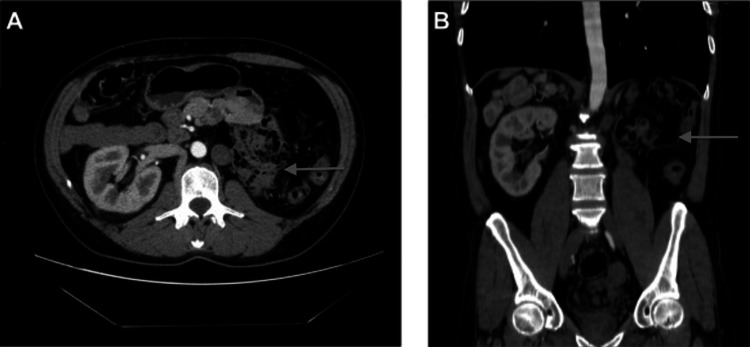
(A) Axial CT imaging with left renal agenesis. (B) Coronal CT imaging with left renal agenesis.
